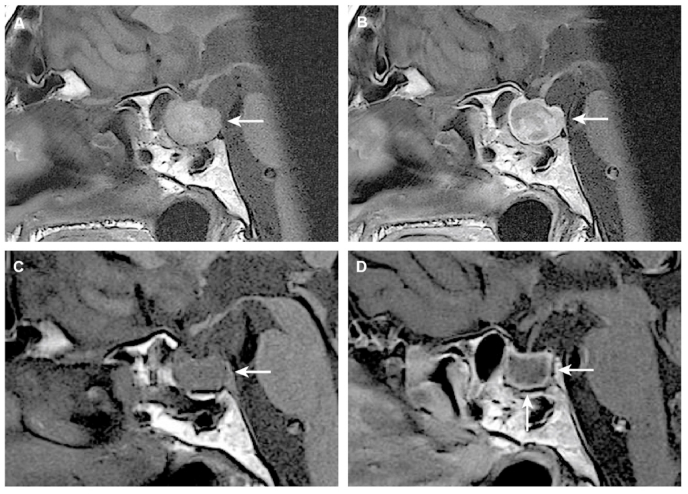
Follow-up magnetic resonance imaging (MRI) scans. MRI performed at the one-month follow-up (A, B) shows partial regression of the sellar lesion. Partial regression of the high signal intensity on T1-weighted MRI (A) and heterogenous, mostly peripheral contrast enhancement (B) is noted, consistent with aging of the blood breakdown products and partial liquefication of the hemorrhage. MRI performed at the one-year follow-up shows chronic, liquefied hematoma with T1-signal characteristics close to water (C) and peripheral contrast enhancement (D).
